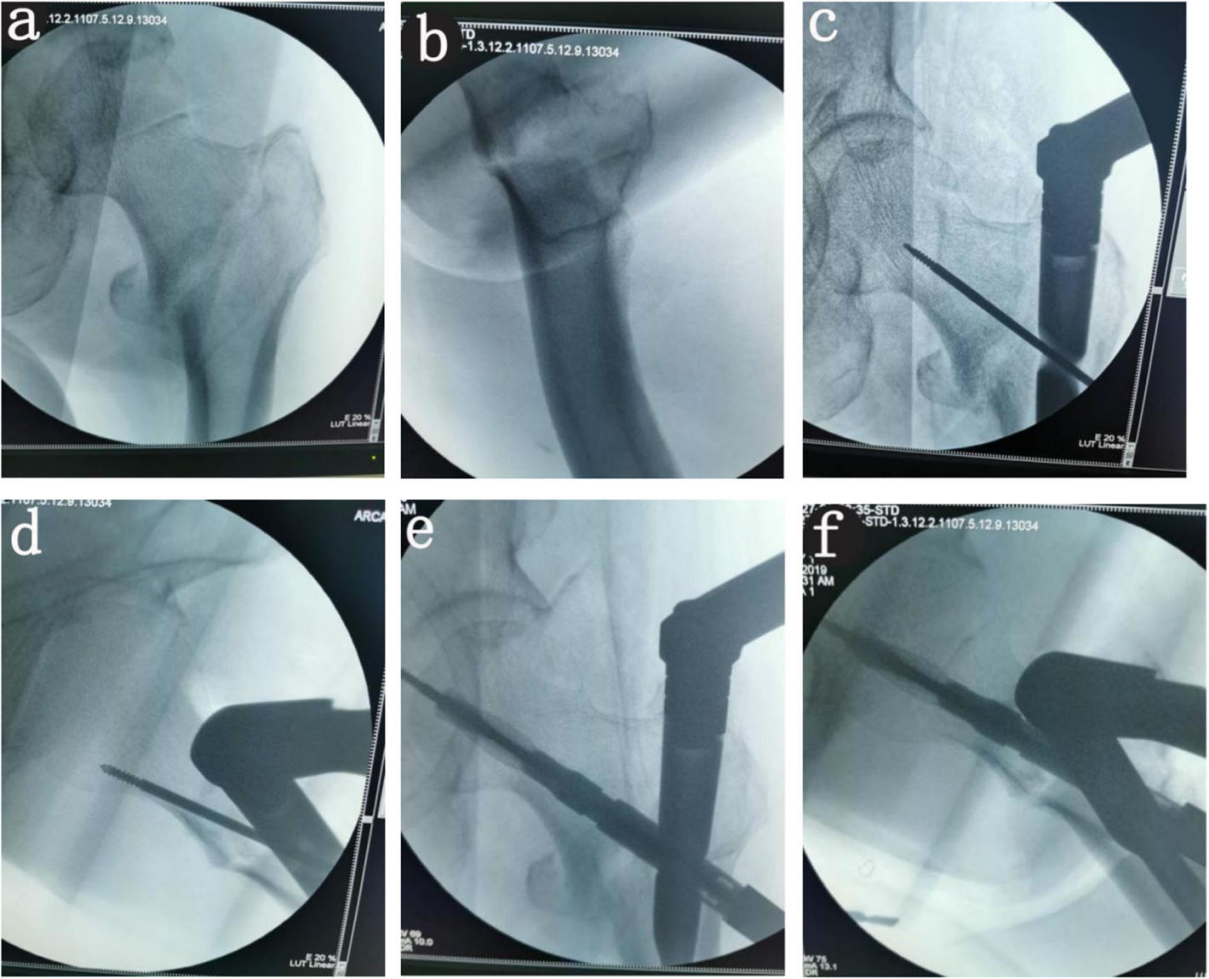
Female, 87 years old, left femoral intertrochanteric fracture caused by fall at home (AO A2.1). Surgical treatment was performed 5 days after admission. The femoral neck screw was implanted by routine locating techniques. a Preoperative X-ray examination (AP posture). b Preoperative X-ray examination (lateral position). c Intraoperative anteroposterior fluoroscopy shows that the guide needle was in the middle of the femoral neck. d Intraoperative lateral fluoroscopy shows that the guiding needle was in the anterior of the femoral neck. e Perspective anteroposterior position after placing the spiral blade. f Perspective on the posterior lateral position when inserting the spiral blade shows the blade in the anterior of the femoral neck
